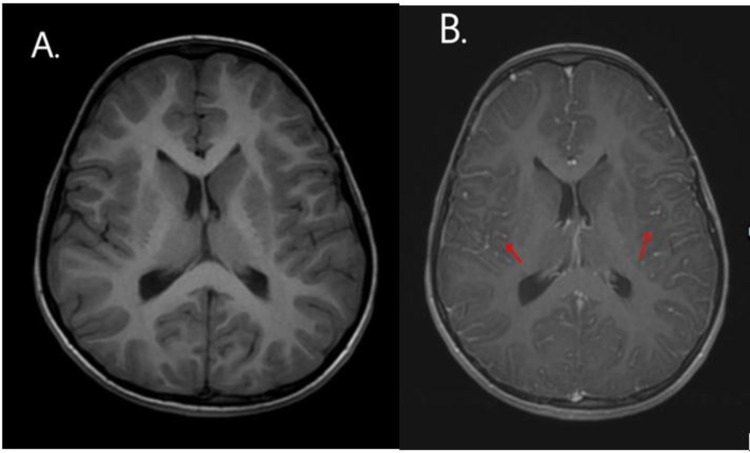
Axial T1-weighted MRI of a six-year-old boy with COVID-19 encephalopathy (A) without contrast - normal and (B) with contrast - diffuse leptomeningeal involvement in bilateral cerebral hemispheres COVID-19: coronavirus disease 2019
